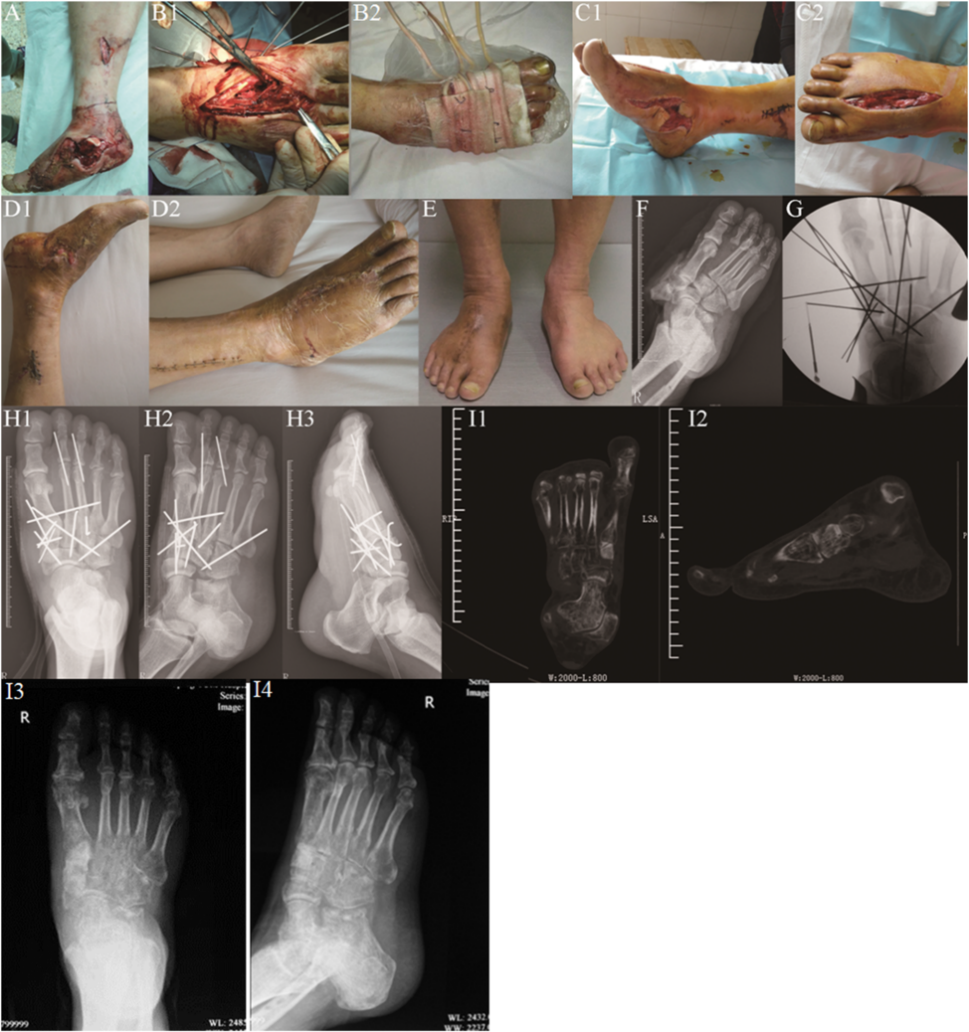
Male patient, aged 55, with right foot severe open Lisfranc fracture-dislocation, was admitted to hospital 2 h after being crushed under heavy objects. One-stage internal fixation using k-wires associated with VSD was performed during emergency. a Severe open wound on the right foot with Lisfranc fracture-dislocation and badly contaminated, combined with ankle fracture shown on X-ray film. b One-stage reduction and multiple k-wire internal fixation were performed via the open wound and dorsal incision, sealed with VSD kit. c The condition of original wound and incision was checked when VSD kit was changed 5 days after surgery. d The patient was discharged from hospital 23 days after surgery, when the dorsal incision recovered well after suture, and the original open wound also recovered well after postage stamp grafting. e One year after injury and 8 months after weight-bearing walking, with satisfying appearance and function. f On X-ray film before surgery, fracture-dislocation was observed to the right Lisfranc joint, combined with distal fibula fracture. g Multiple k-wire cross-fixation was performed during surgery; the fracture-dislocation reached to anatomical reduction under X-ray. h AP, oblique, and lateral X-ray showing the condition of fracture-dislocation after one-stage internal fixation with good alignment. i Space broadening was not observed by CT review and X-ray film half a year after removing internal fixators
